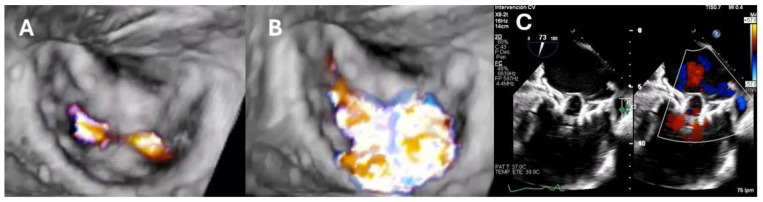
Transcatheter mitral valve replacement after M-TEER. Transesophageal echocardiography interventional study: (A): 3D imaging of the mitral valve apparatus in diastole. Clip device placed in the A2–P2 position. (B): 3D imaging of the mitral valve apparatus in systole. Mitral regurgitation grade 4+ consisting of two main jets (lateral and central). (C): 2D and color Doppler imaging after clip removal and mitral valve replacement performed with Tendyne (Abbott Vascular, Plymouth, MN, USA).
